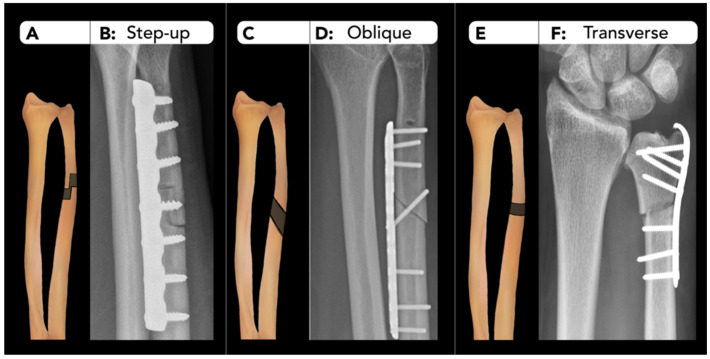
Ulnar shortening osteotomy. (A–F) Various techniques of ulnar shortening osteotomy. (A,B) The step-cut approach, which involves an interlocking step pattern at the osteotomy site, resulting in a larger contact surface area and enhanced rotational stability. (C,D) The oblique approach, characterized by an angled cut across the ulna, allowing for gradual compression and control shortening. (E,F) The transverse approach, featuring a horizontal cut perpendicular to the ulna’s long axis, which simplifies the surgical procedure and provides straightforward alignment during fixation. Corresponding radiographs with fixation hardware for each technique are also shown.
